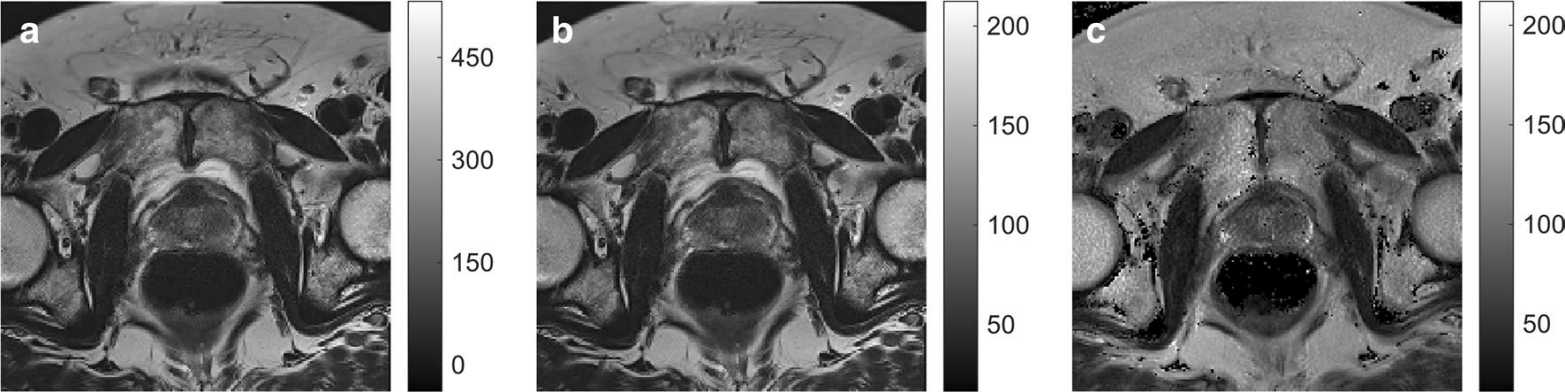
a Original bias field corrected T2-weighted image (T2WI), b AutoRef_FH_ pseudo-T2 map and c multi-echo spin echo (MESE) T2 map for one volunteer. The original T2WI and the pseudo-T2 map were window levelled from their minimum to maximum image intensity, while the MESE T2 map was kept on the same level as the pseudo-T2 map ([16 ms, 212 ms]). The T2WI was registered to the MESE image space before normalization
